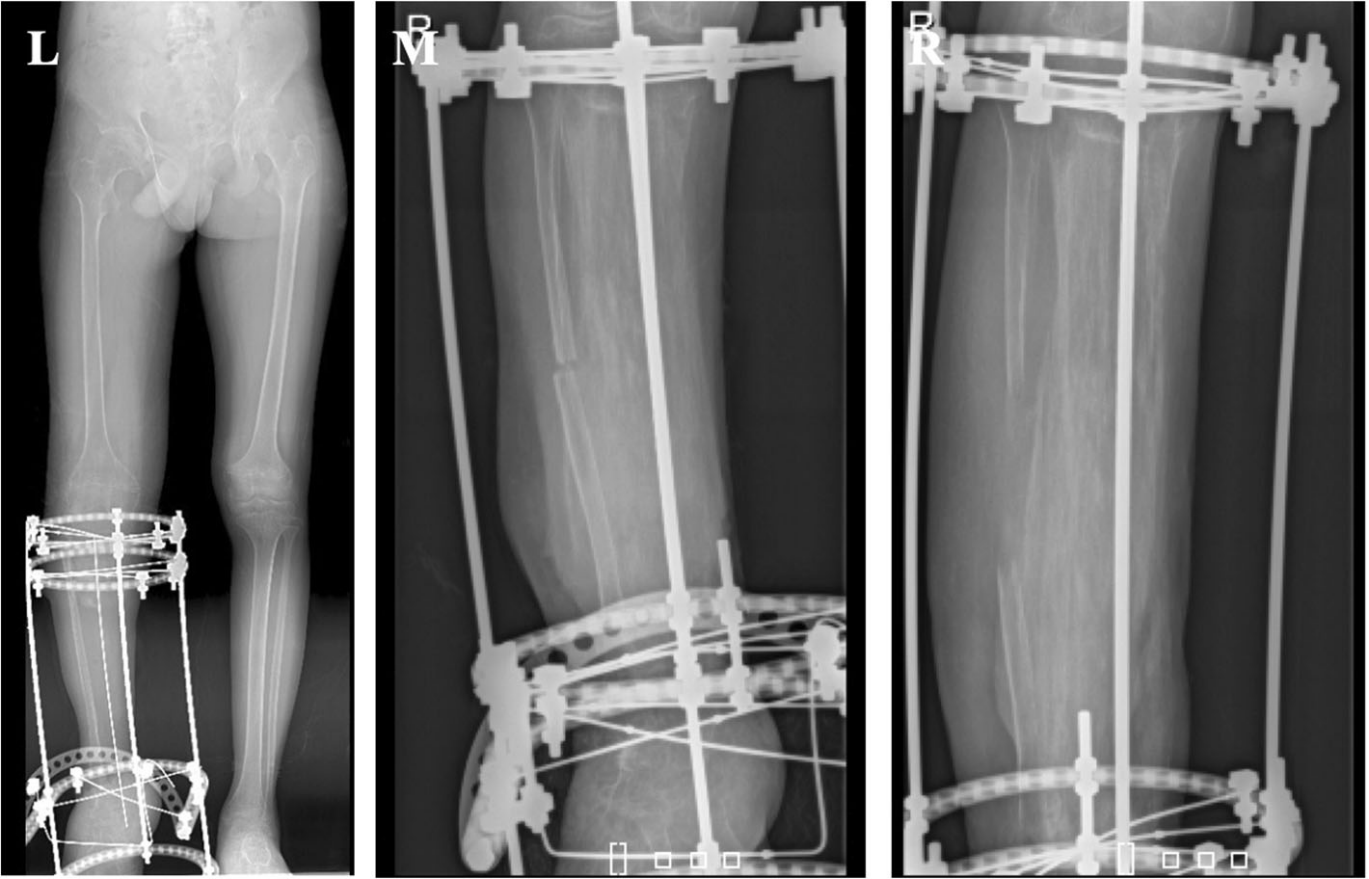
Left, X-ray of bilateral lower limbs showing limb discrepancy. Middle, 10 days post-op fibular osteotomy. Right, 45 days post-op fibular osteotomy
